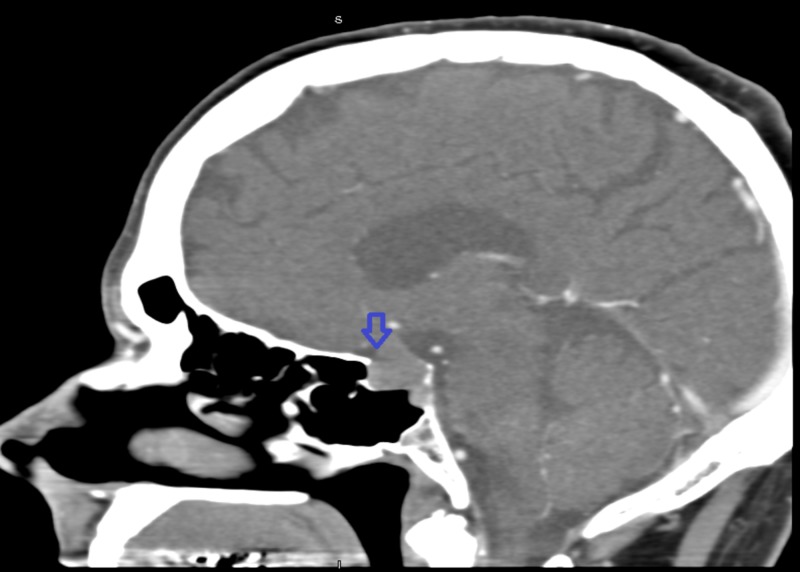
Computed tomography of the head with arrowhead pointing towards the pituitary macroadenoma
